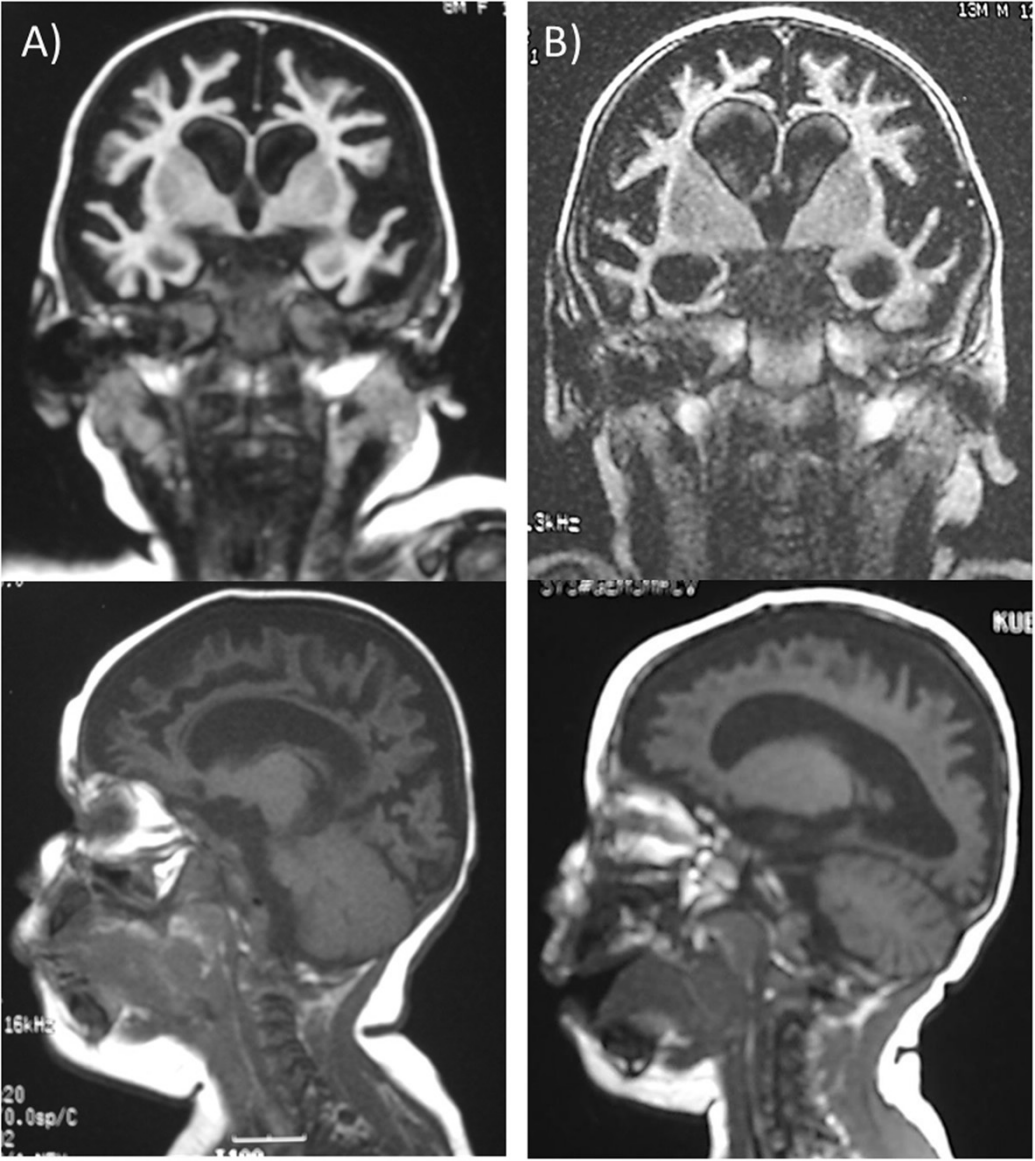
Coronal and sagittal views of T1-weighted MRI-head of ARCA patients demonstrating diffuse cortical atrophy and ventriculomegaly. A) V-10 at 8 months of age, B) V-14 at 10 months of age.
